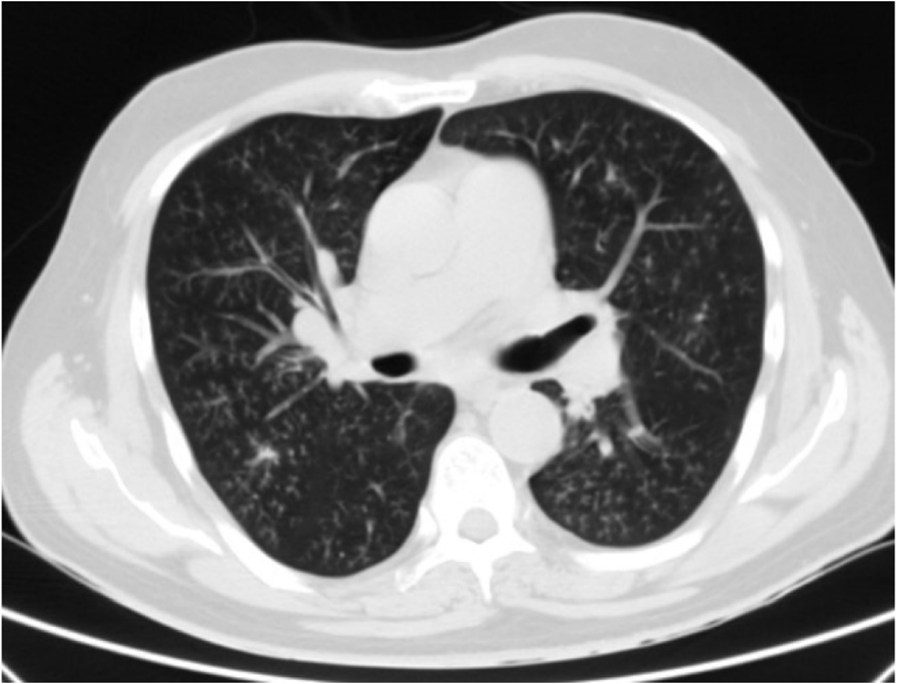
Infectious bronchiolitis: thin section CT shows diffuse clustered branching tree-in-bud opacities
